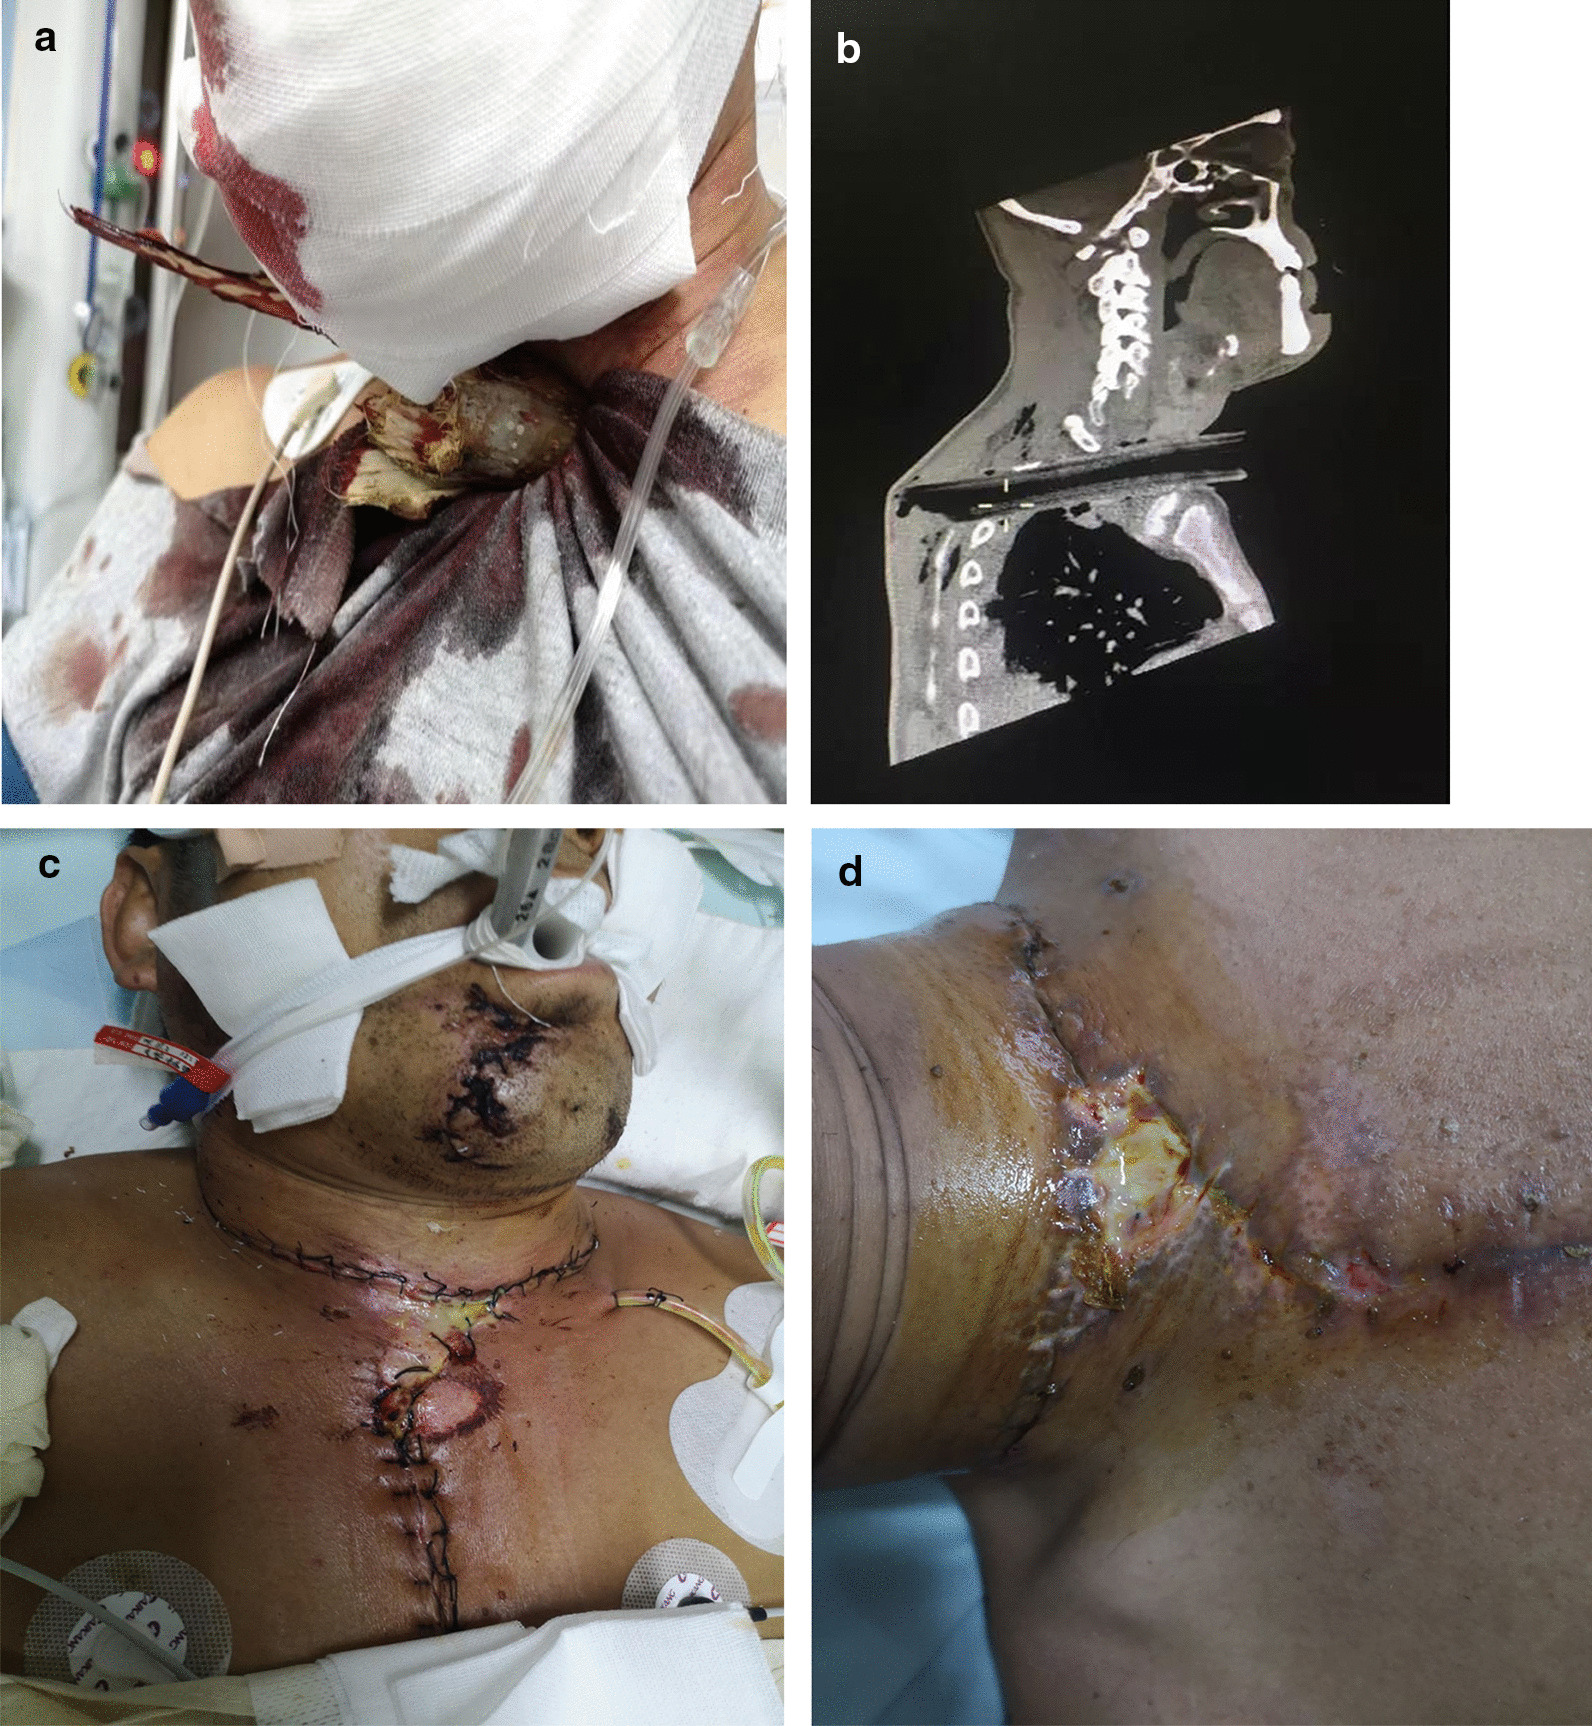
a The patient was stabbed by a thick branch penetrating from the neck to the chest and back. b The CT imaging data of the patient. c The dressing was changed, and the wound condition was observed (2019.11.07). d The wound condition of the patient after the suture was removed (2019.11.21)
